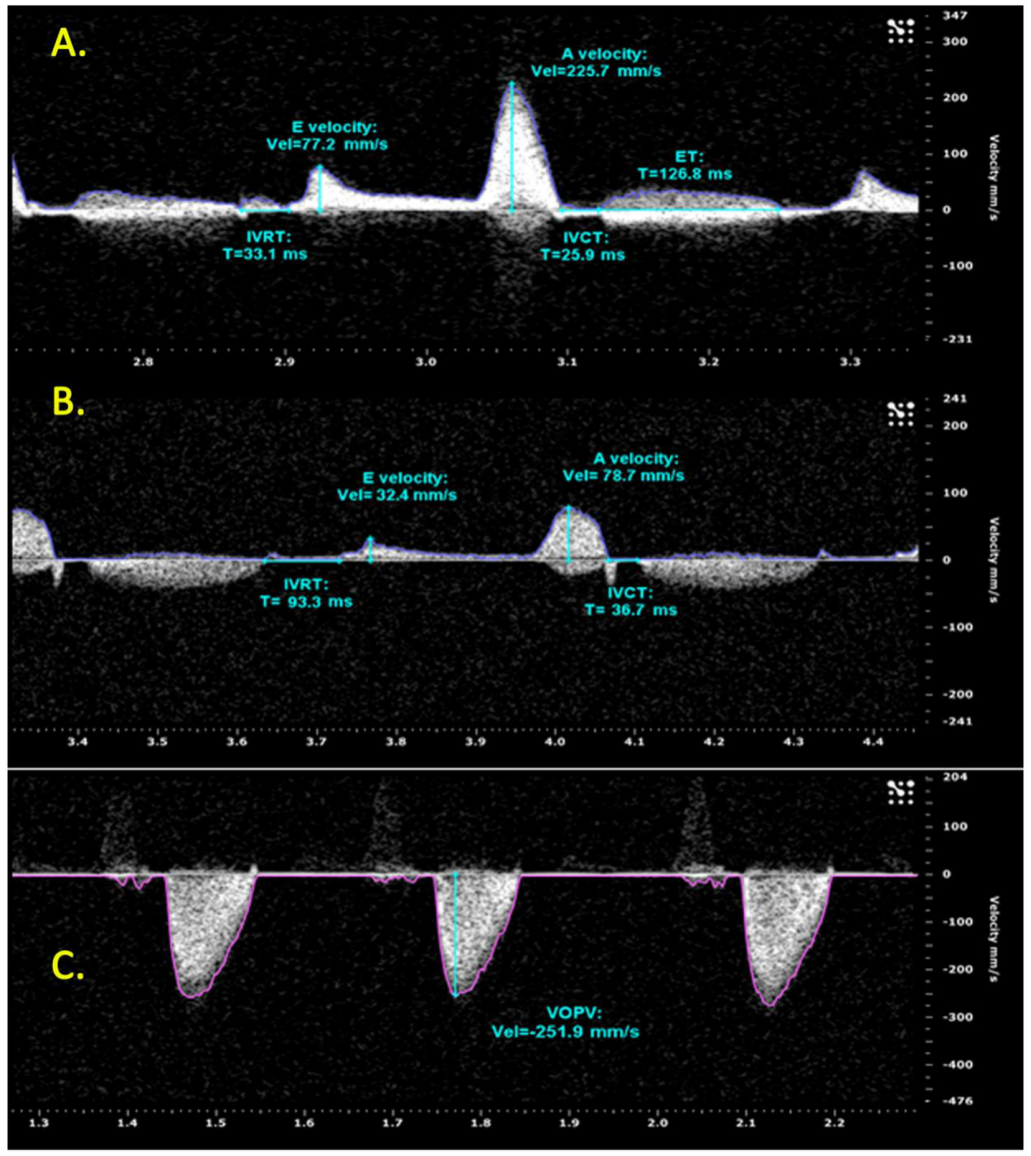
Pulse wave Doppler image of ventricular inflow and outflow velocity. Ventricular inflow image was used to measure IVRT, IVCT, ET, early (e) filling peak velocity and atrial (a) filling peak velocity in a WA ZF at: (A) 28°C and (B) 18°C. Note the difference in x-axis in the 28°C condition and 18°C condition. (C) Ventricular outflow peak velocity (VOPV) of a WA ZF at 28°C. The Y-axis indicates velocity (mm/s) and the x-axis indicates time (ms). ET, ejection time; IVCT, isovolumetric contraction time; IVRT, Isovolumetric relaxation time; Vel, velocity.
